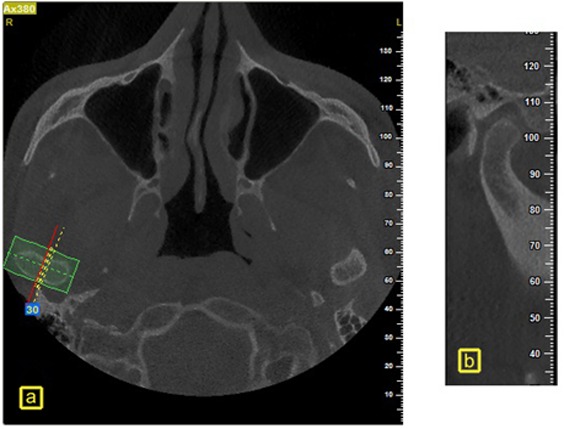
Reconstruction of CBCT sections in a sample case. a: Axial views in which the condylar process had its widest mediolateral diameter, b: Central sagittal section of the condyle
